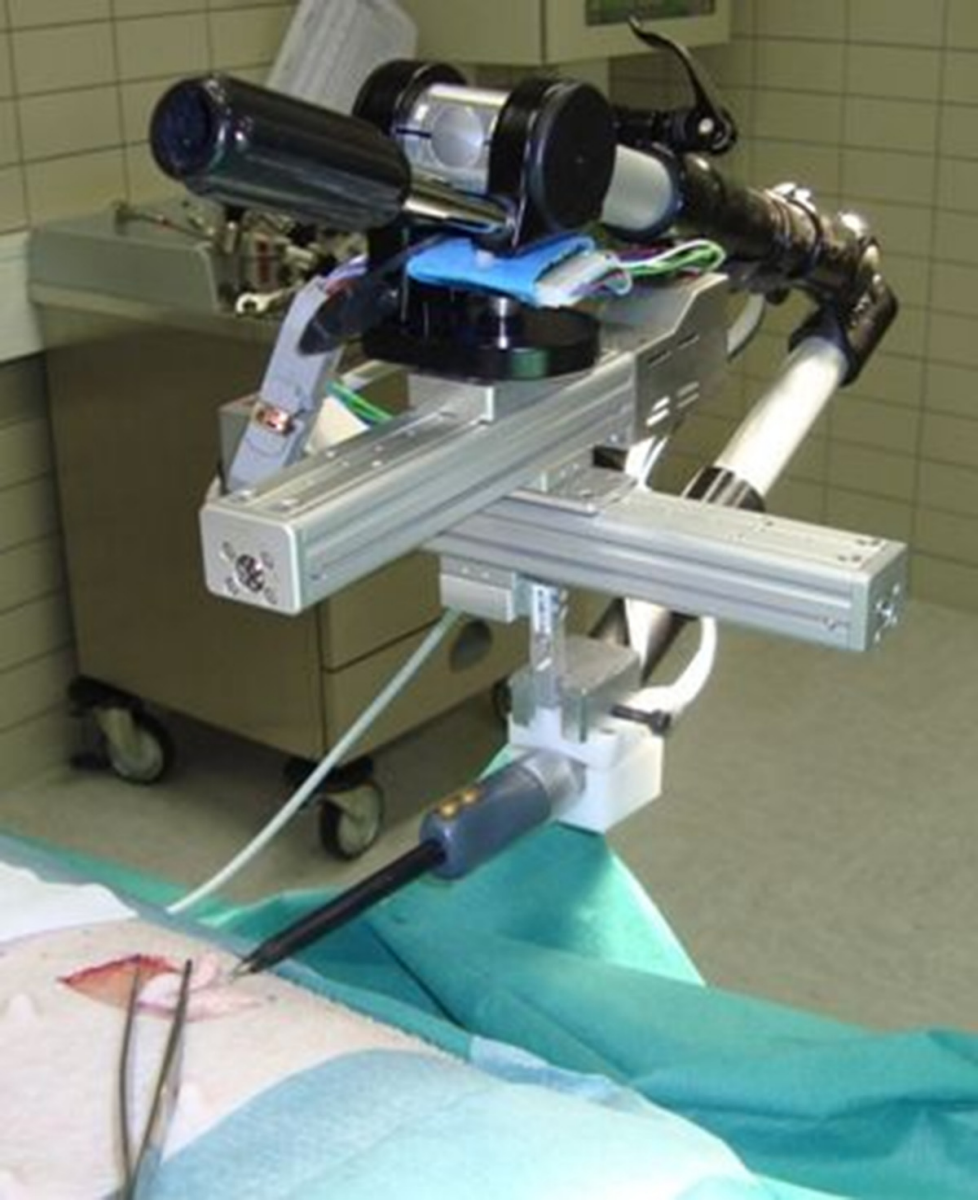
Automatic device for soft tissue dissection. Self-constructed apparatus fixed at the operating table and loaded with ultrasonic scalpel. The instrument can be moved engine-driven into two directions (aluminium tracks).
